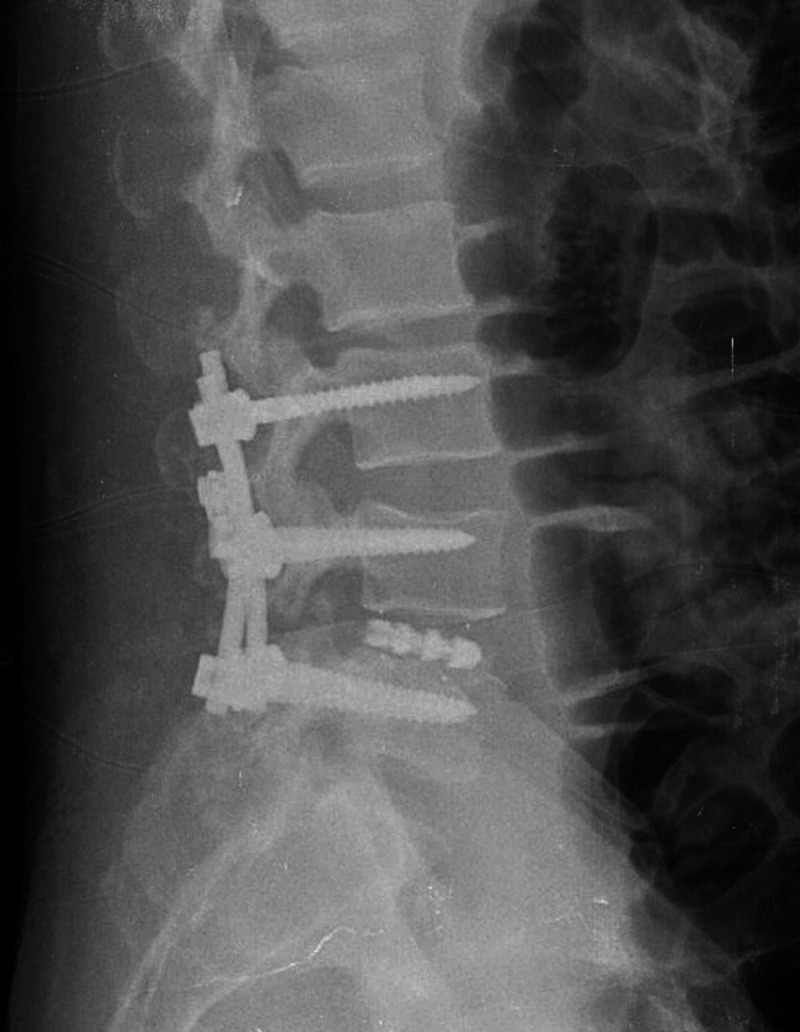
Postoperative (three months follow-up) standing roentgenogram of the lumbar spine showing excellent sagittal balance and pedicle screw-rod fixation in situ.
